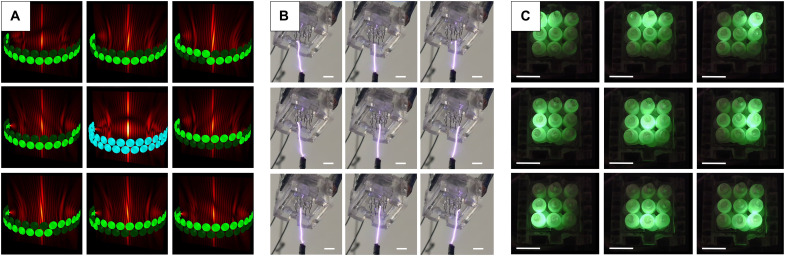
Activating specific neon bulbs by targeting a 3 by 3 array. (A) Ultrasonic emitters and generated amplitude field. (B) Plasma spark targeting individual electrodes. (C) Illumination of the corresponding neon bulbs. Scale bars, 1 cm.
